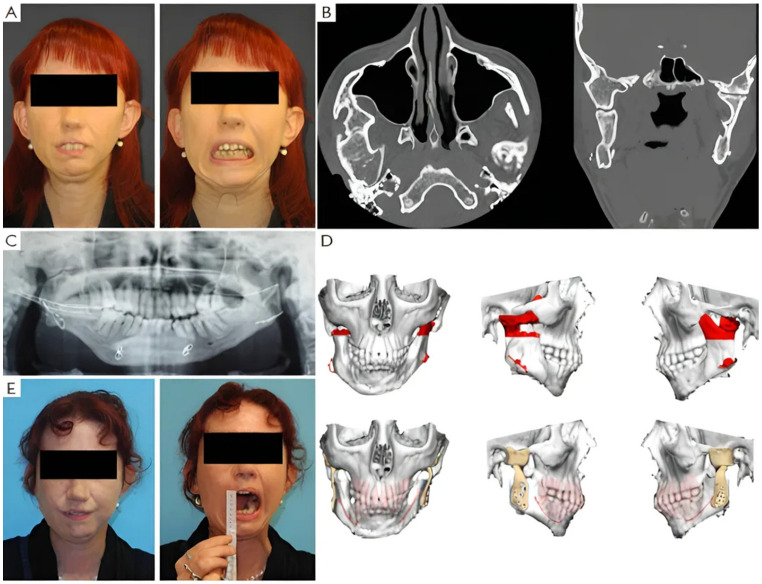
Clinical case of TMJ ankylosis with previous costochondral graft. (A) Clinical frontal preoperative view and preoperative maximum interincisal opening of patient (less than 1 cm). (B) Axial and coronal TC scans show bilateral ankylosis. (C) Postoperative orthopantomography shows mandible reconstruction with costochondral graft. (D) Virtual planning of the resection and virtual planning and design of the prosthesis. (E) Postoperative clinical view and postoperative maximum interincisal opening of patient at 1 year follow up (3 cm).
